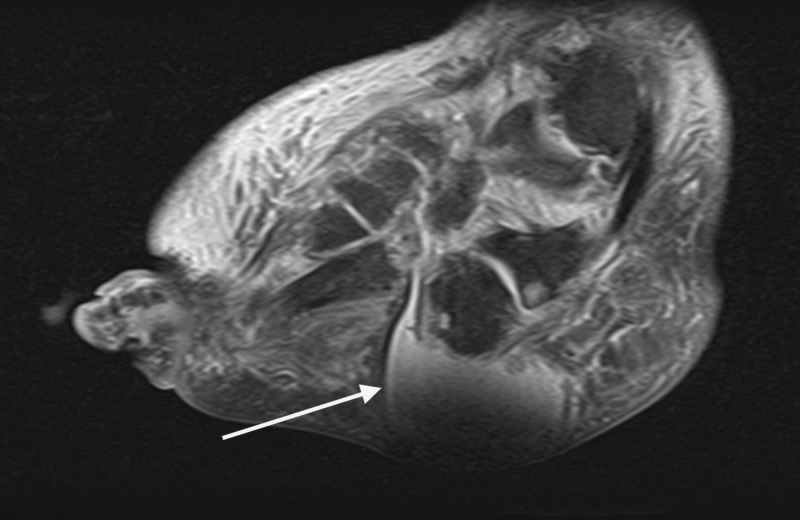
Sagittal MRI revealed well-organized fluid filled lesion within the plantar lateral tissue that is communicating with the disrupted joints of the midfoot.
